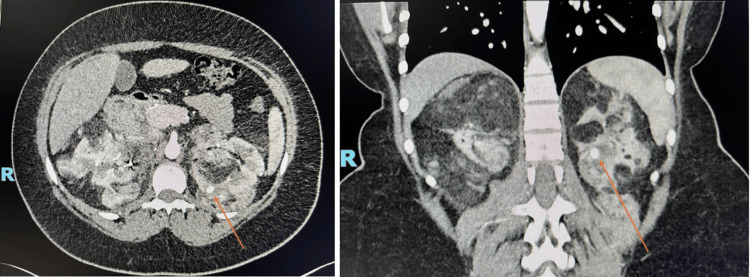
Follow-up CT imaging: arterial phase with coronal and axial views of the kidneys The arrows highlight a left 9 mm intra-tumoral aneurysm for prophylactic embolisation due to high risk of rupture. This is the hyperdense circular abnormality.
